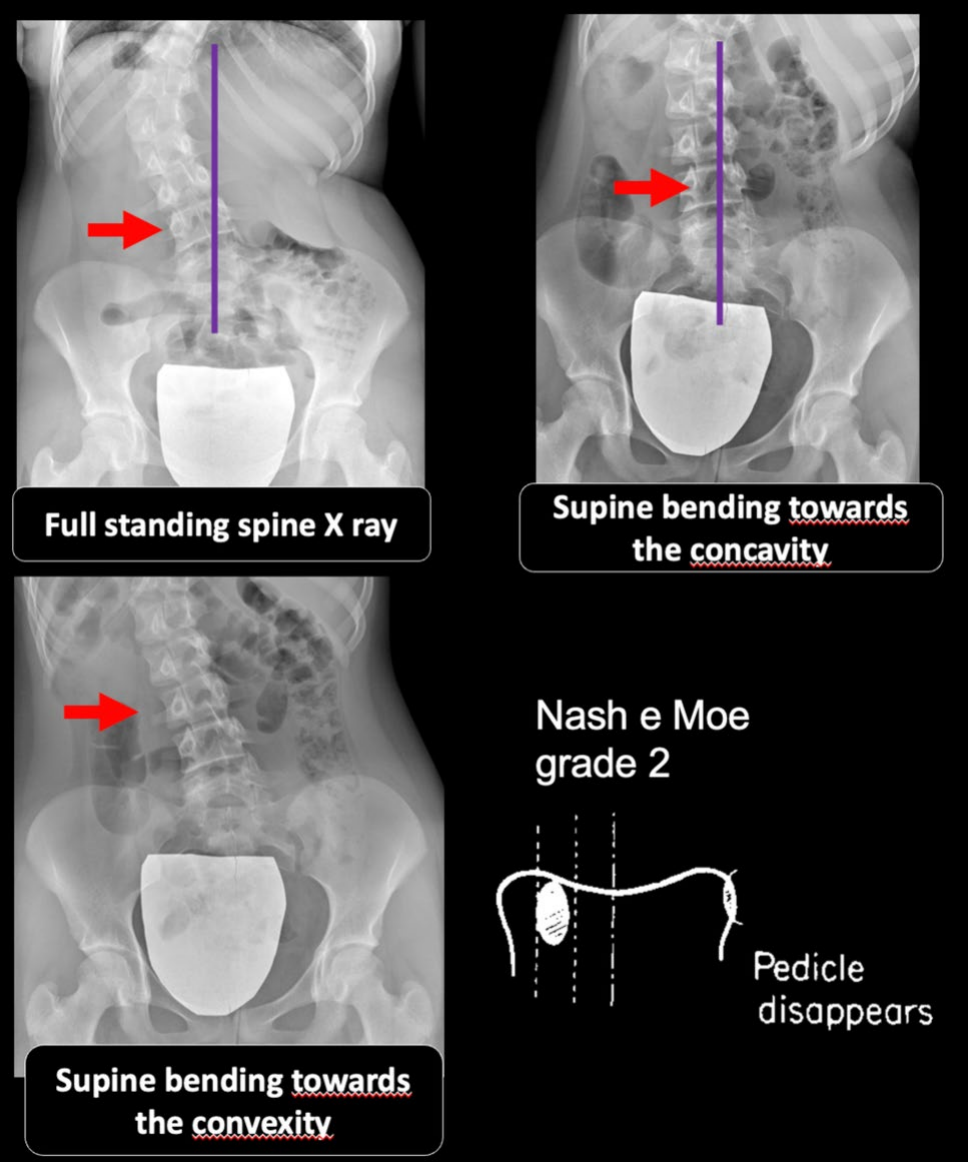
Criteria for LIV selection of L3 based on supine bending X-rays in TL/L curves according to Kim et al. and Shao et al.
